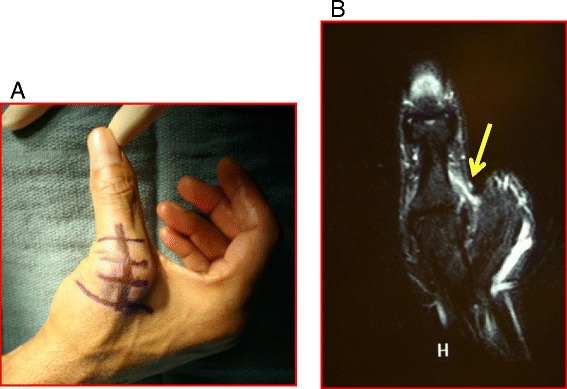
a Preoperative photograph demonstrating a patient with no endpoint to valgus stress testing of the thumb MCP joint. b A T2-weighted coronal sequence demonstrating a complete tear of the UCL which is detached from the proximal phalanx (*yellow arrow*)
